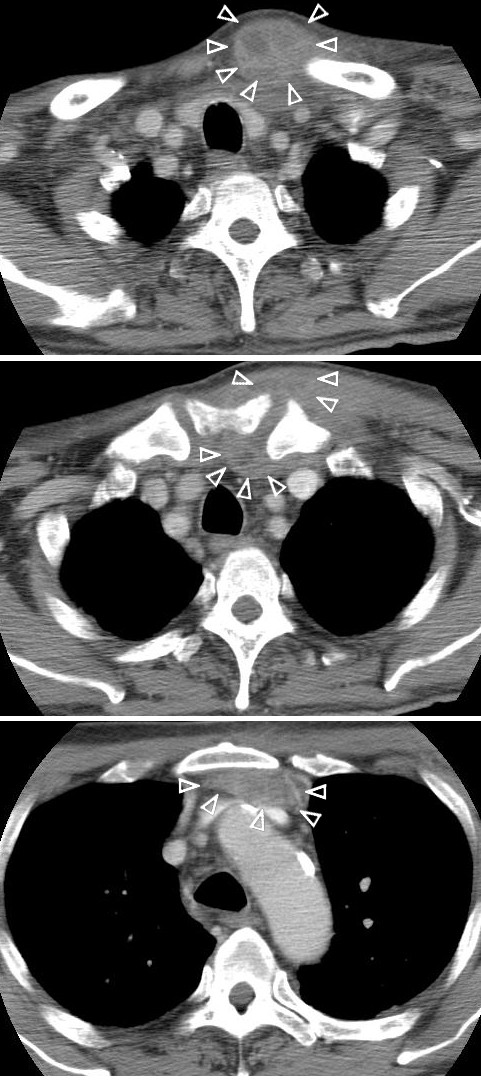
Thoracic CT scan: A CT scan using intravenous contrast shows an abscess ( *allow head: △* ) around the left SCJ (A and B). The abscess is compartmental structure. The rim of the mass is slightly enhanced, but the center of the abscess is not enhanced. The abscess extends to the left sternocleidomastoid muscle (A) and retrosternal region (C).
